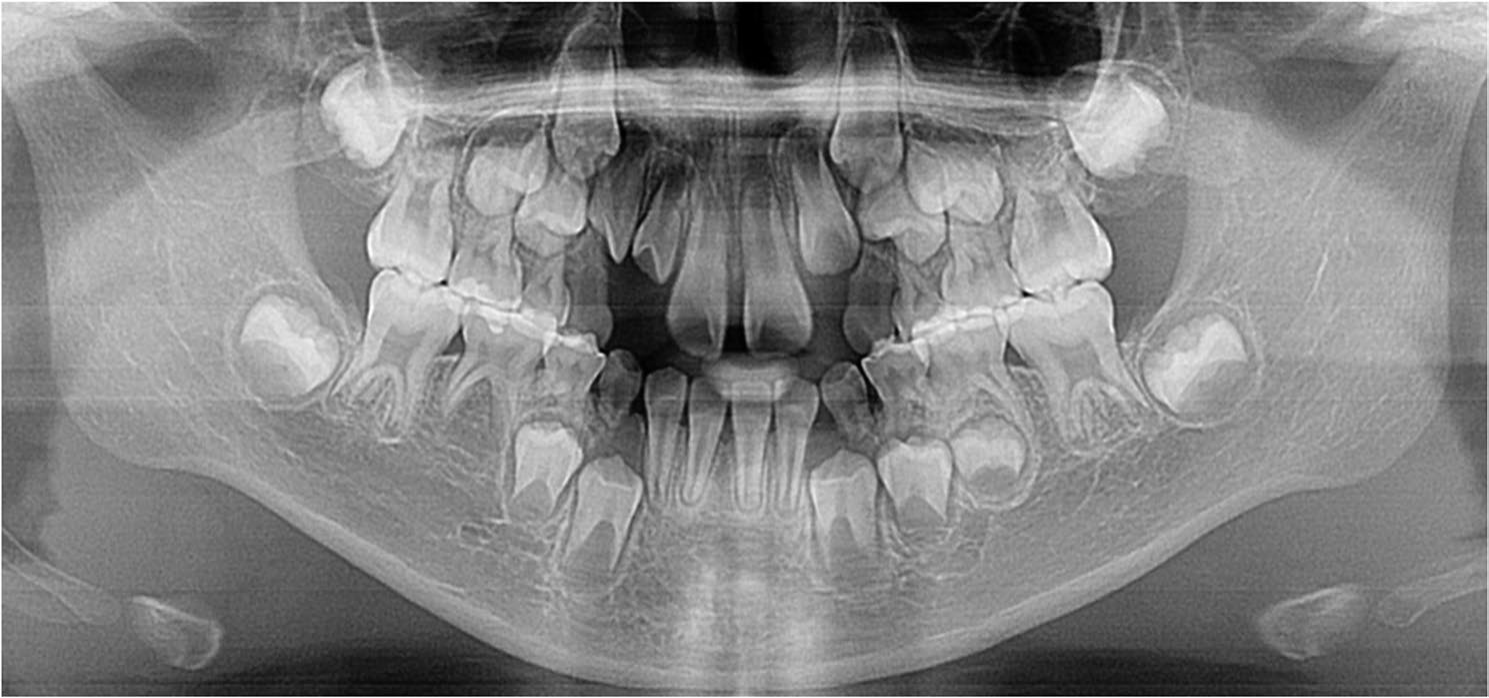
Panoramic radiograph of a 6-year-old female showing congenitally missing right mandibular second premolar, 1 supernumerary tooth located in right maxillary lateral incisor region
